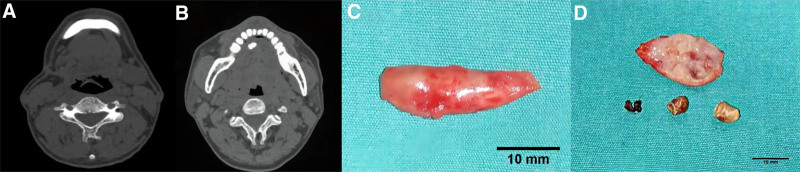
(A) Computed tomography (CT) shows the absence of a right submandibular gland in the patient 1. (B) CT shows 2 irregular high-density masses in the front part of the main submandibular duct in the right mouth floor area of patient 1. (C and D) Stones and suture were taken out during the operation of patient 1.
